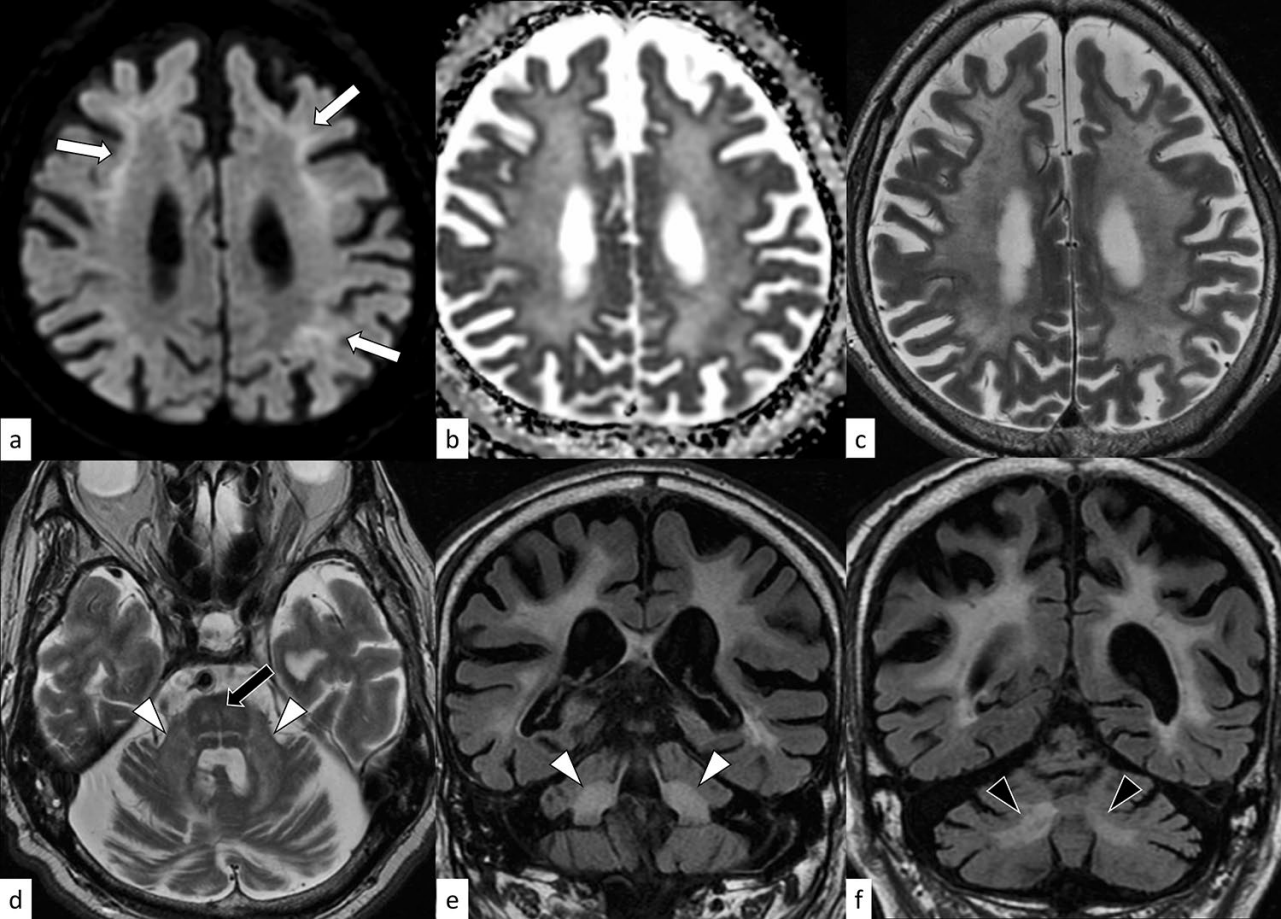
A 79-year-old man with FXTAS. DWI shows hyperintensity bands along the corticomedullary junction (a, arrows). ADC map (b) and T2-weighted axial images (c) show diffuse white matter hyperintensity with atrophy. The middle cerebellar peduncle sign (d, white arrowheads) and hot cross bun sign (d, black arrow) are observed on T2-weighted axial image. FLAIR coronal images show the middle cerebellar peduncle sign (e, white arrowheads) and paravermal hyperintensity (f, black arrowheads)
